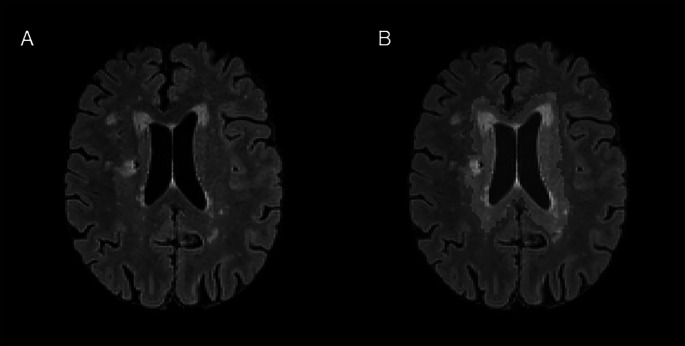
White matter hyperintensity classification. Periventricular and deep white matter hyperintensities (WMHs). A T2-weighted FLAIR image for a representative participant is displayed in A. The periventricular mask (yellow) and deep mask (red) are overlaid on the T2-image in B
